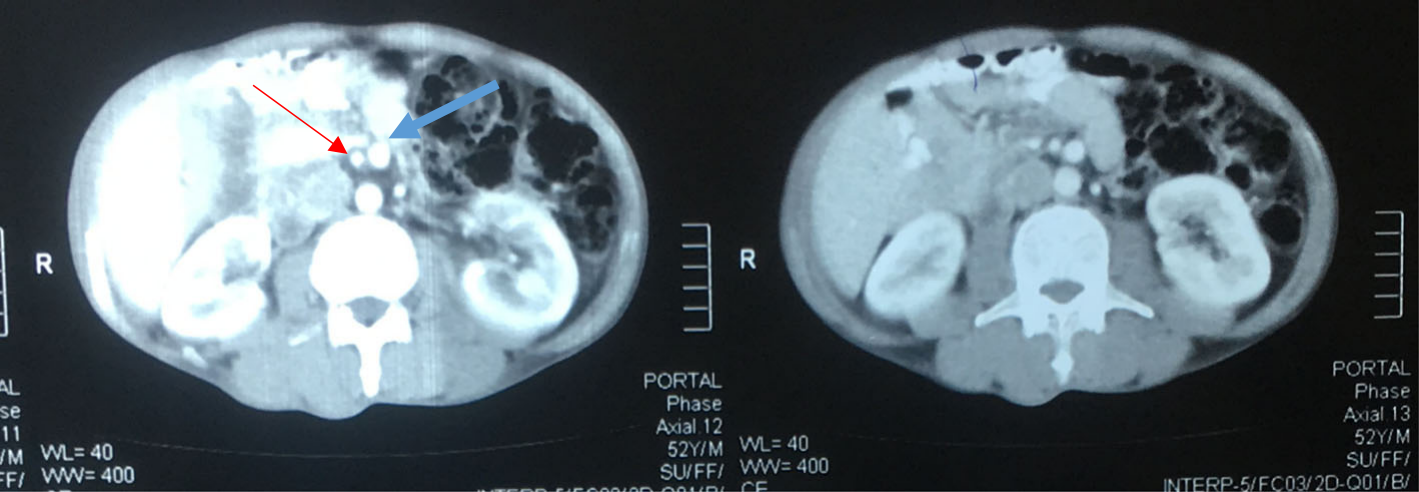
Computed tomographic scan of the abdomen showing inversion of superior mesenteric artery (red arrow) and superior mesenteric vein (blue arrow)
